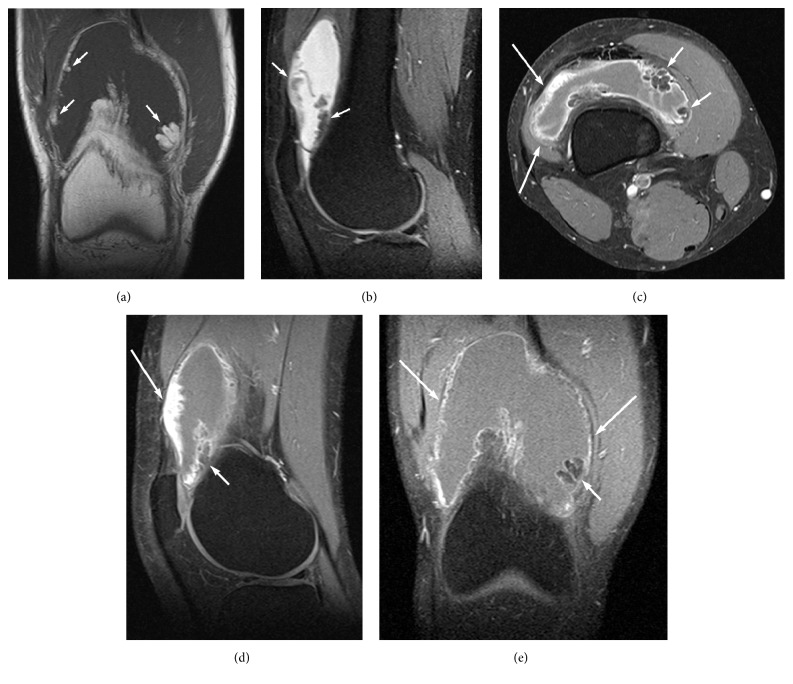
MR images on coronal T1-weighted (a), sagittal PD-weighted with fat saturation (FS) (b), and axial, sagittal, and coronal T1-weighted with FS after iv administration of gadolinium (c), (d), and (e), respectively, depict frond-like villi projecting inwards from the synovium with signal equal to fat on all imaging sequences (short arrows). After iv administration of gadolinium, enhancement of the overlying synovium is observed (long arrows), but no enhancement of the underlying fat is detected. Joint effusion is present distending the joint capsule, without any sign of menisci or ligaments' injury, or osseous erosions.
